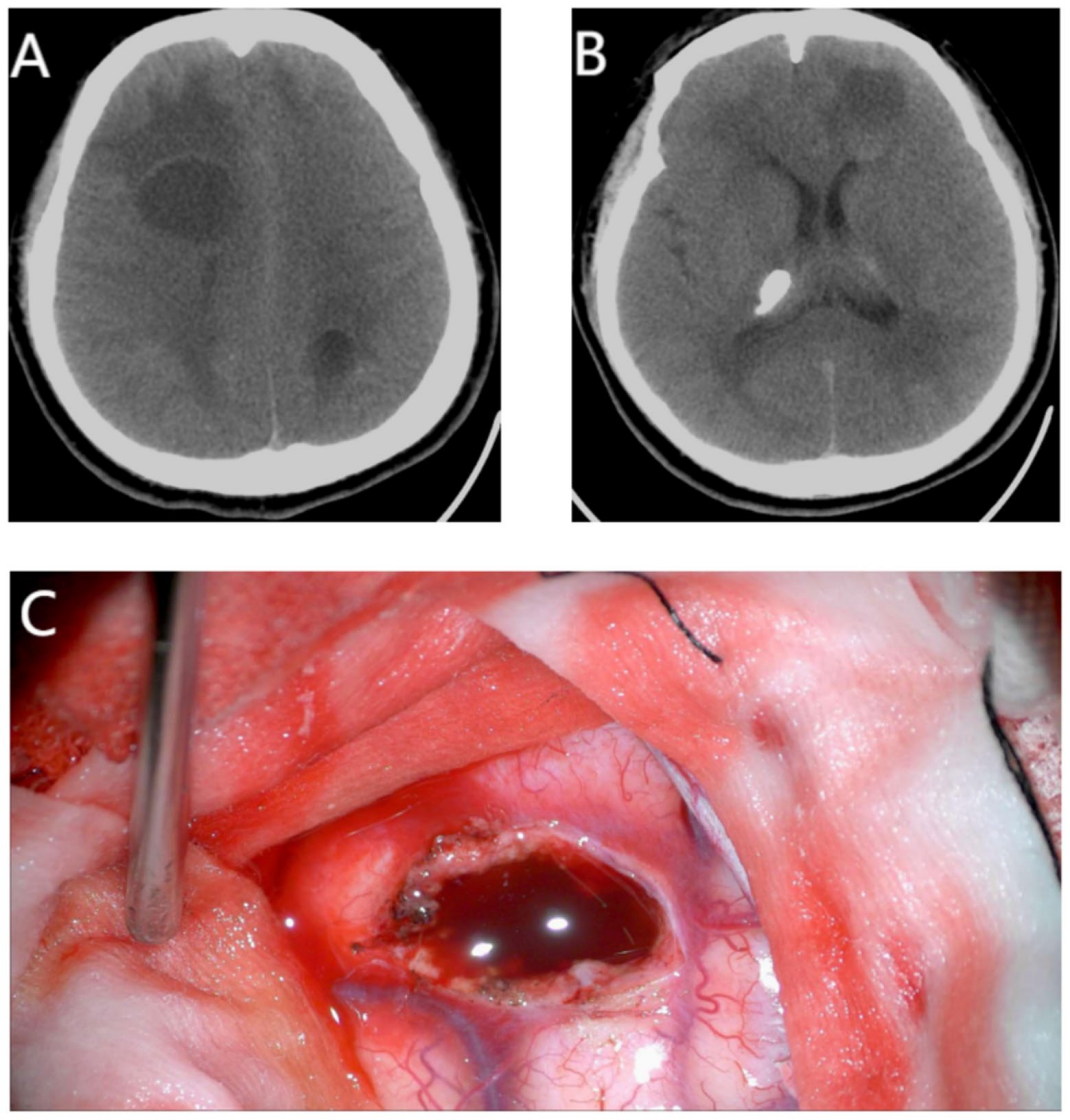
Preoperative cranial CT and intraoperative right frontal lobe cystic lesion of patient in April 2019. (A,B) Preoperative cranial CT showed cysts in bilateral frontal lobe and left parietal lobe, calcification of right thalamus. (C) Lesions in right frontal lobe with cyst fluid.
